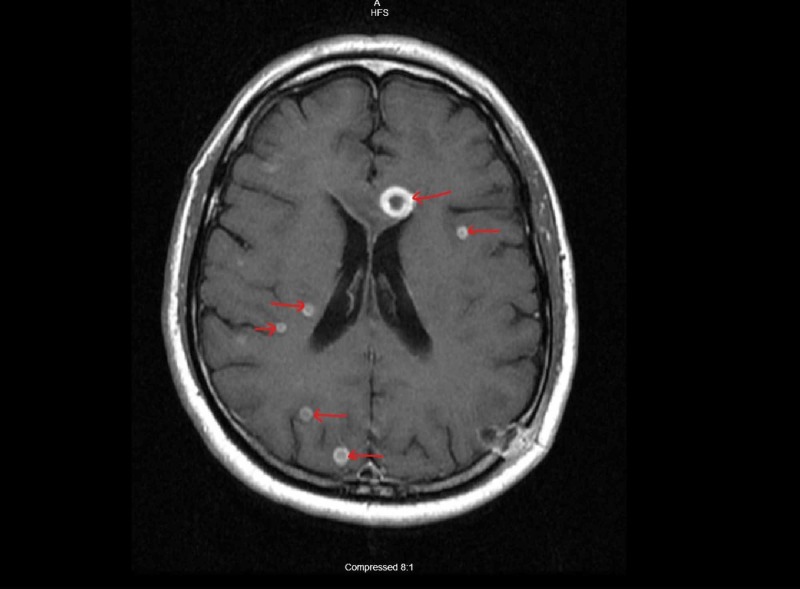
MRI brain with IV contrast showing ring enhancing lesions in cerebral cortex, one lesion close to ventricle (Arrows pointing)
